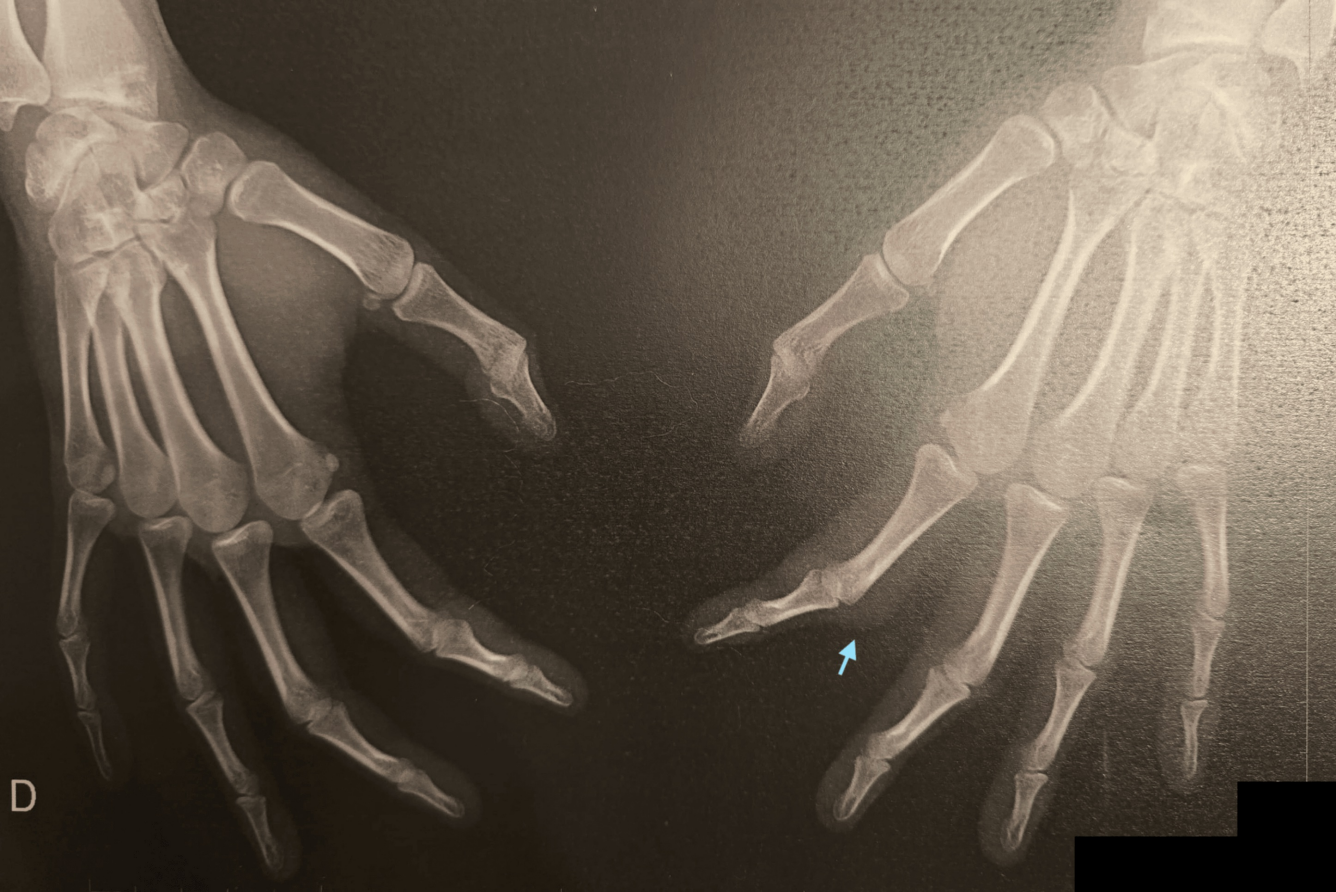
Plain radiographs of both hands with light blue arrow marking an evident soft tissue thickening without bony abnormalities
